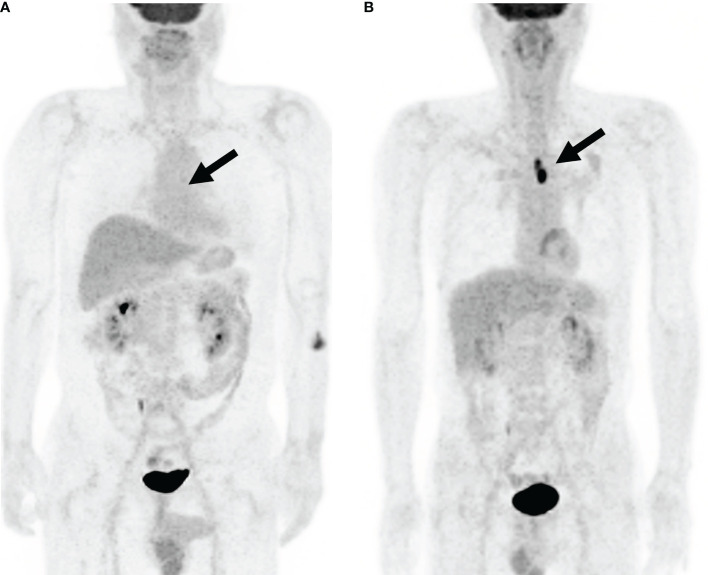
Representative cases of upstaging and downstaging according to SUVmax Maximal intensity projection images of FDG PET/CT demonstrate representative cases of upstaging and downstaging. (A) A 73-year-old male patient with ht low SUVmax of 1.0 (red arrow). Thus, he was downstaged into the new proposed stage I. DFS duration was 1,554 days, and OS duration was 2,546 days. (B) A 75-year-old male patient with esophageal SqCC of stage I. The tumor was clearly demonstrated in the image due to its high SUVmax of 16.0 (red arrow). Thus, he was upstaged into the new proposed stage II (Figure 5b). DFS duration was 329 days, and OS duration was 496 days.
